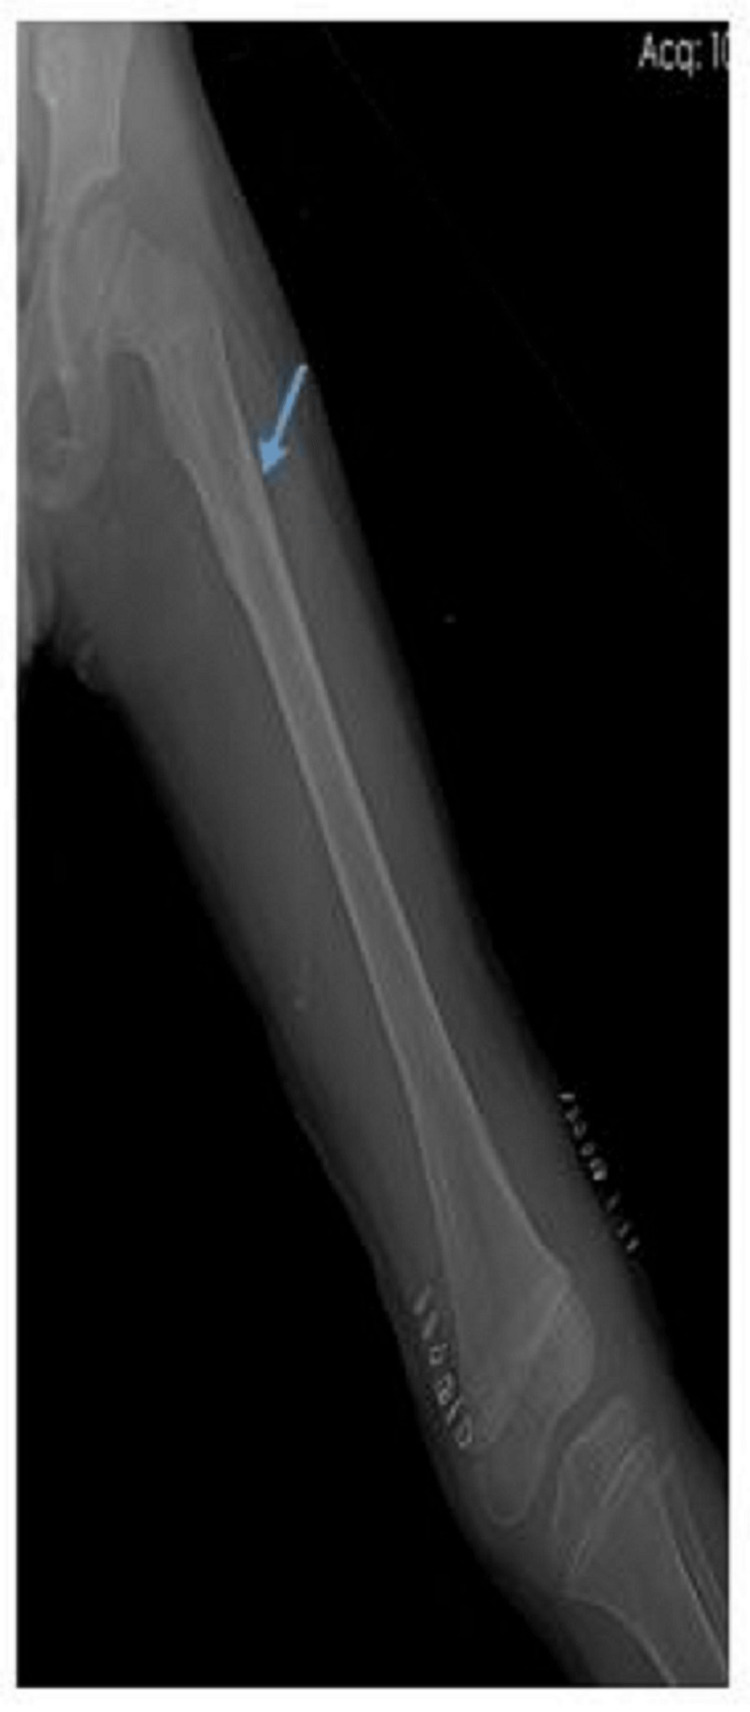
Antero-posterior radiograph of a 9-year-old male who underwent implant removal of TENS after fracture union (blue arrow)
